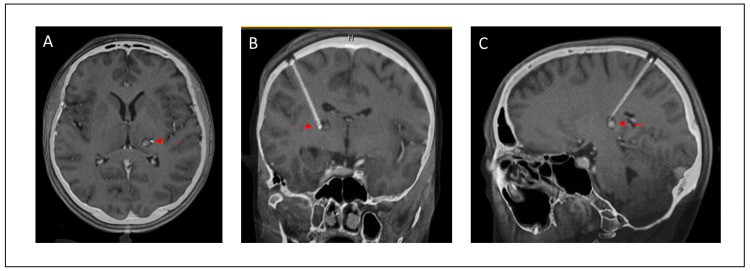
Fusion of the preoperative T1-weighted MRI and intraoperative CT. The preoperative contrast-enhanced T1-weighted MRI sequence in axial (A), coronal (B), and sagittal (C) reconstruction was fused with intraoperative CT showing the catheter which targets the lesion. Red arrows point to the tip of the stereotactically implanted catheter targeting the lesion.
